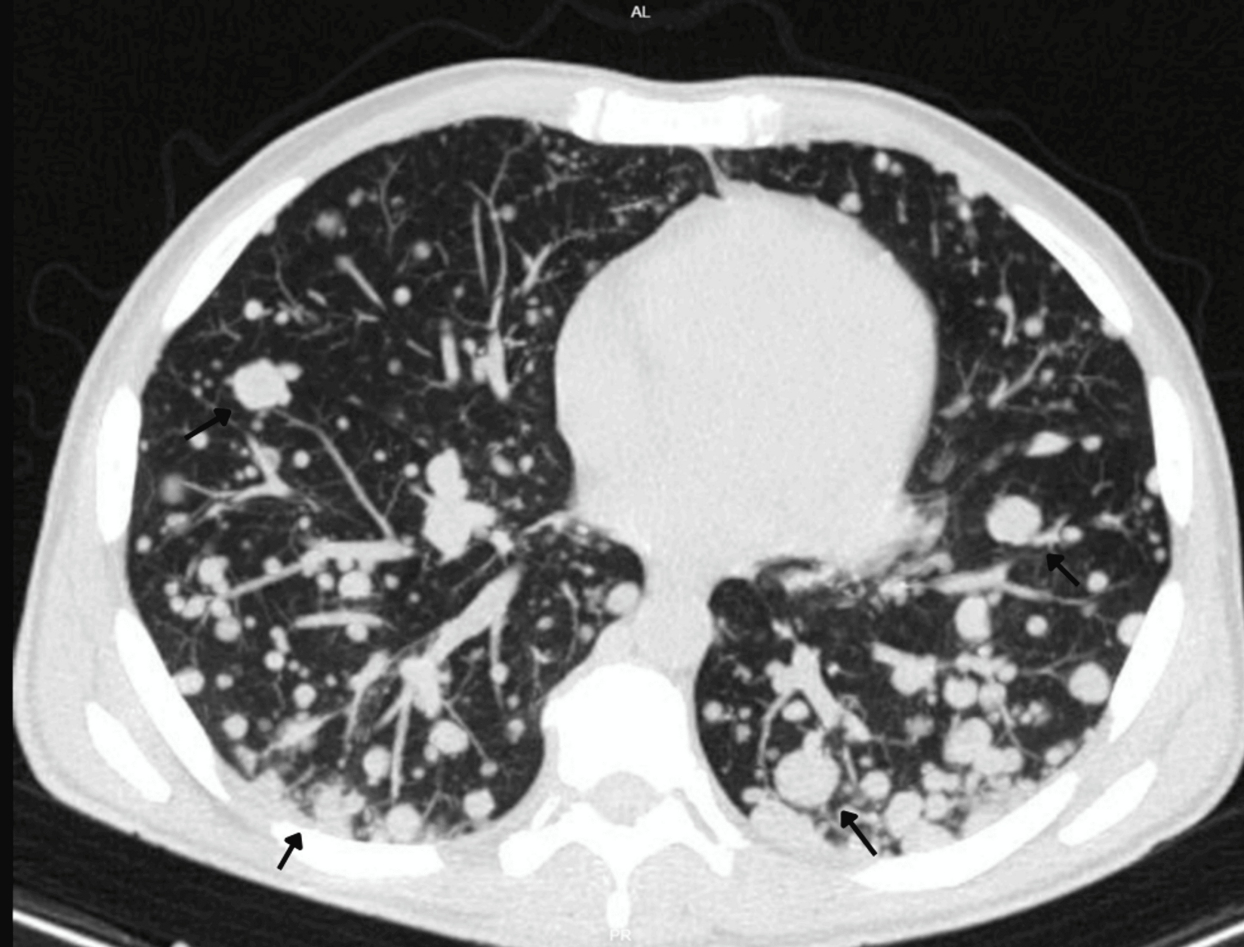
Contrast-enhanced CT of the chest, axial plane. Axial CT image demonstrates numerous bilateral pulmonary nodules of variable size, diffusely distributed throughout both lung fields, several of which show cavitation, consistent with widespread pulmonary metastatic disease. Arrows highlight representative pulmonary metastatic nodules. CT: computed tomography
